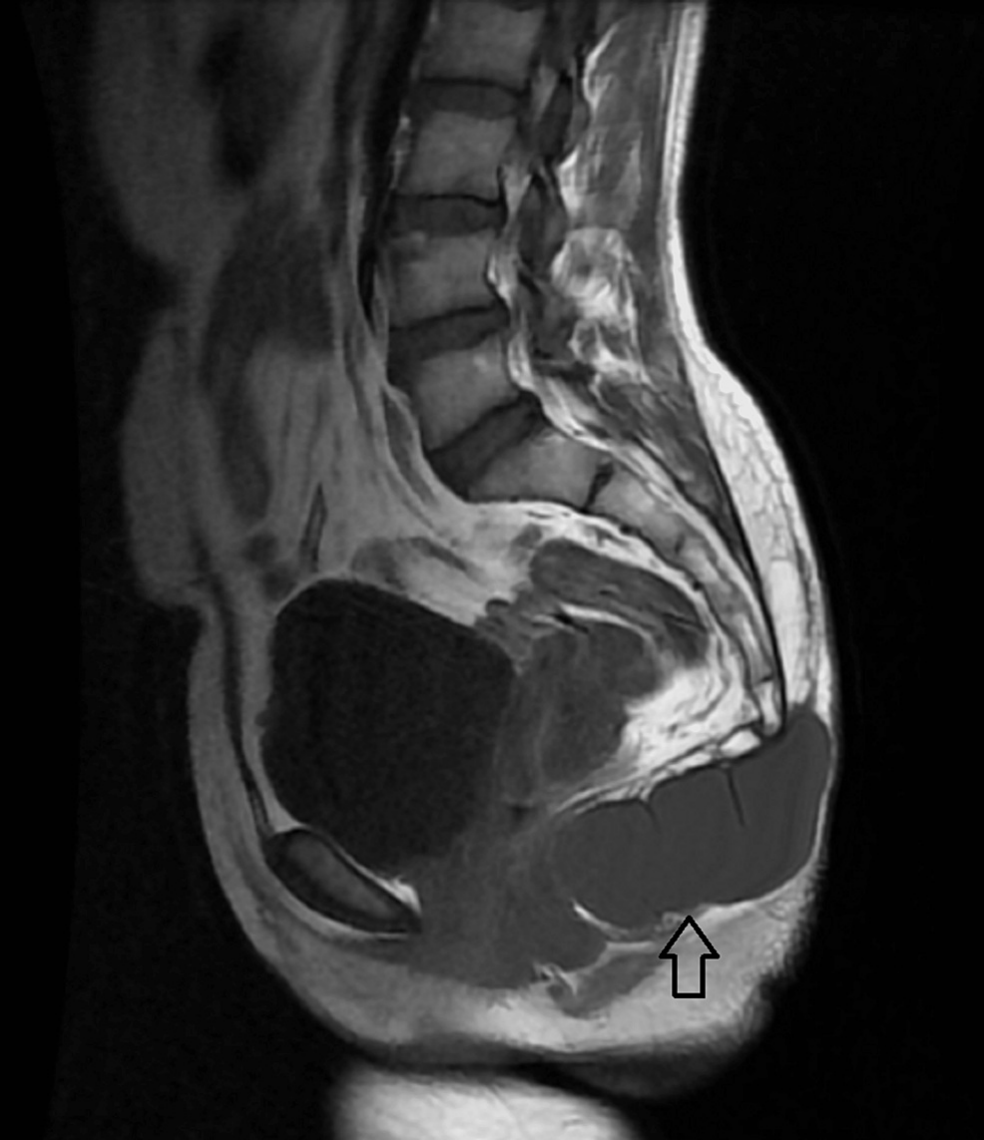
Sagittal T1WI MRI image showing well-defined isointense lesion in the perineal region (black arrow). T1WI: T1 weighted image https://assets.cureus.com/uploads/figure/file/450599/lightbox_8a119400313c11ed9d925f316fd743c0-E2.png
